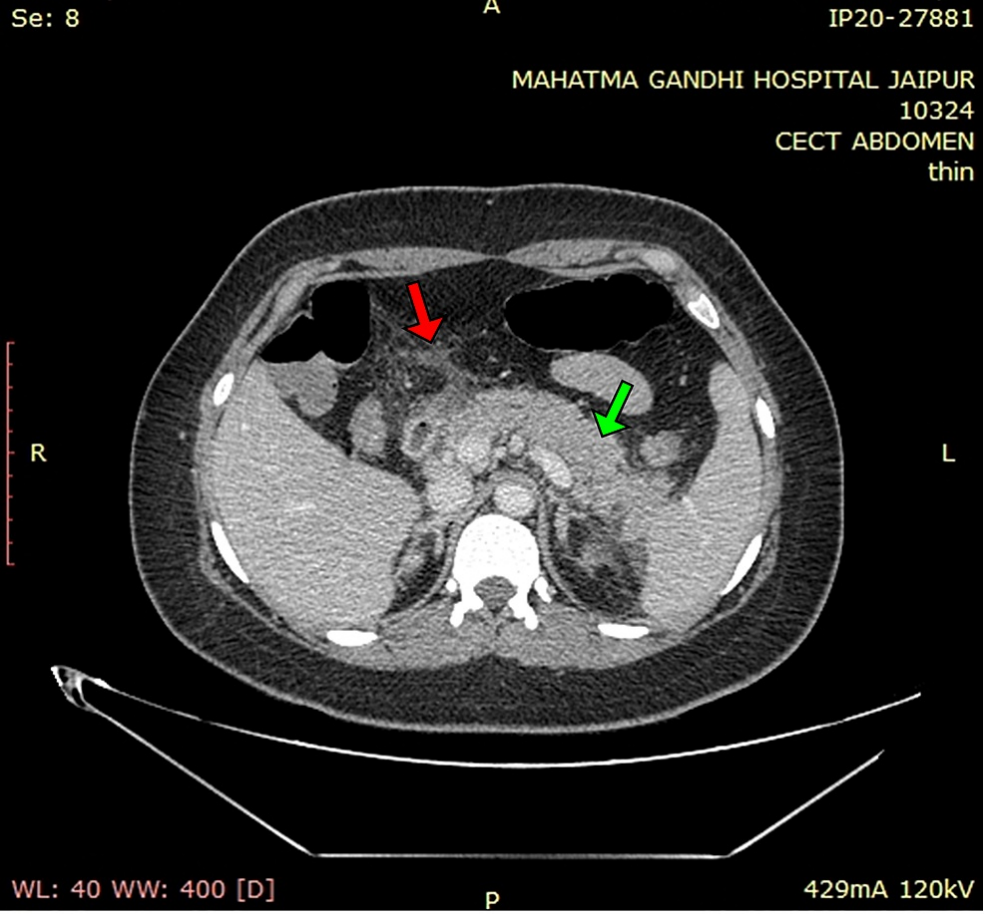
Contrast-enhanced CT of the abdomen (axial section) showing poorly enhancing, bulky pancreas, with no areas of necrosis (solid green arrow), pancreatic duct not dilated, and peri-pancreatic fat stranding (solid red arrow).
